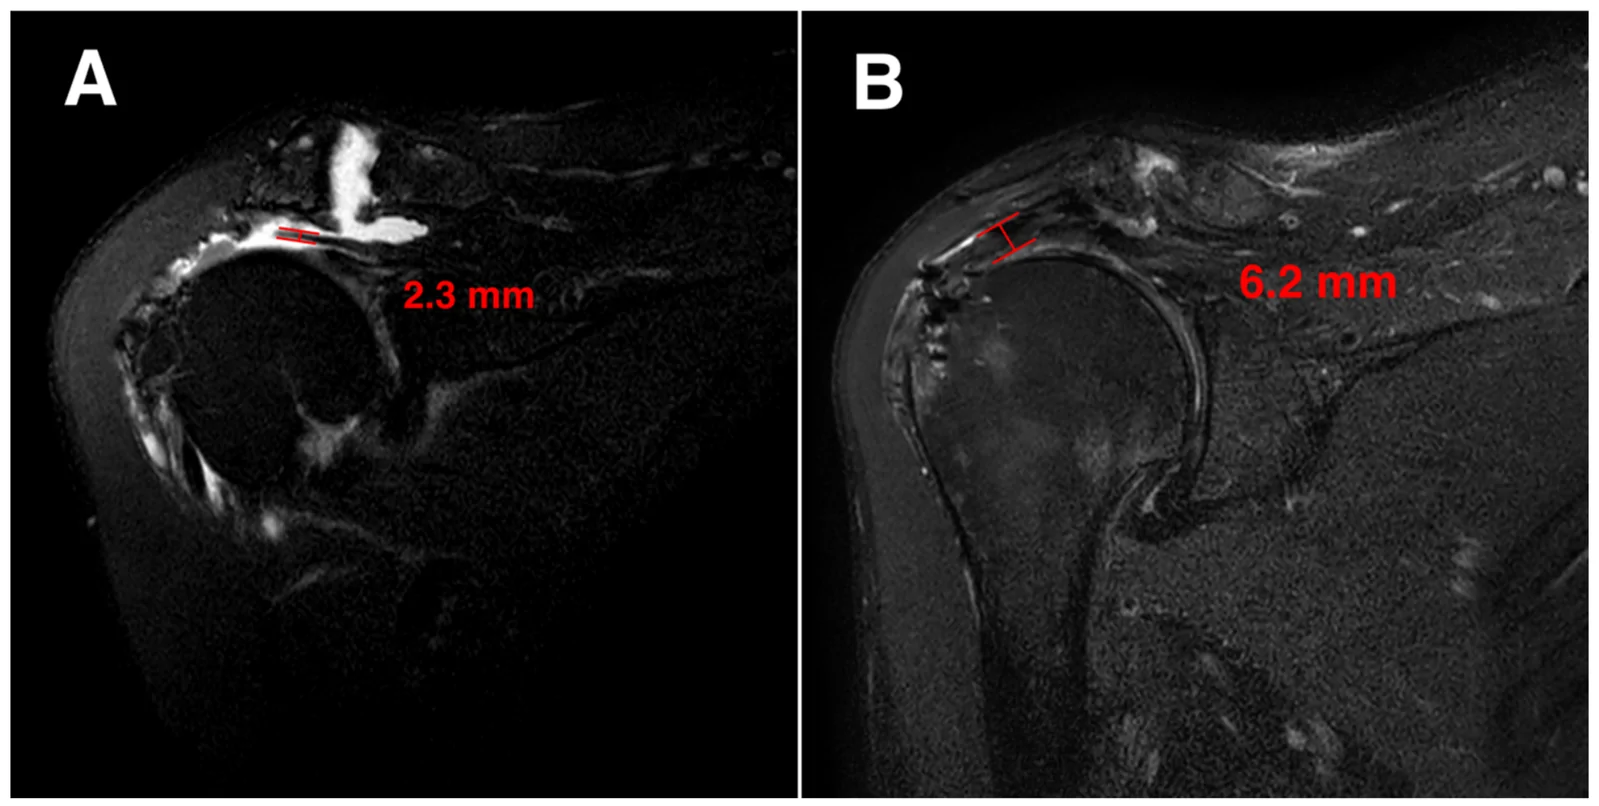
Magnetic resonance imaging of a 67-year-old male with a massive cuff tear of the right shoulder. (A) Preoperative coronal MRI demonstrating a tendon thickness of 2.3 mm at the thinnest portion of the supraspinatus tendon. (B) Postoperative MRI obtained 12 months after surgery demonstrating a healed tendon with increased thickness (6.2 mm) at the corresponding location.
